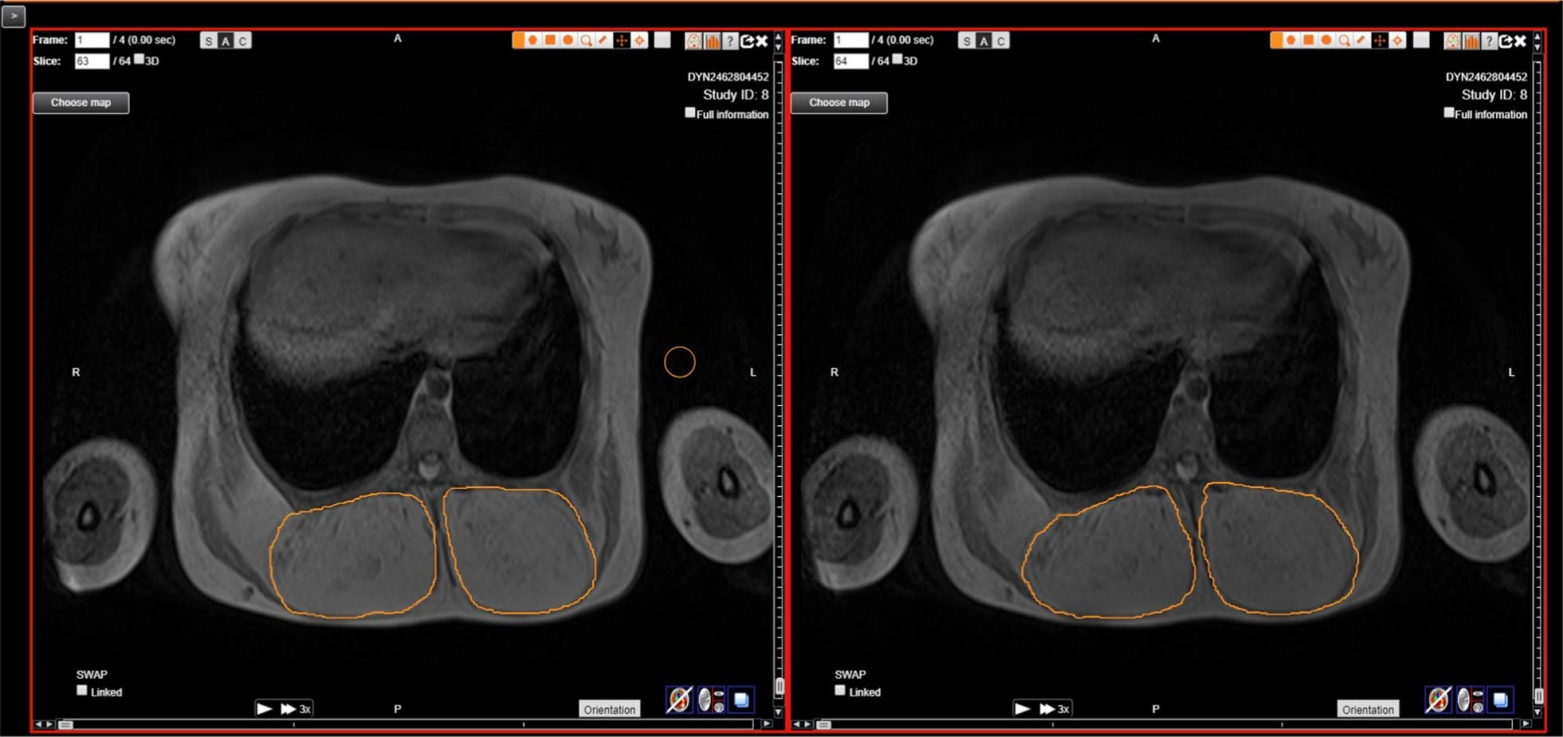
MRI of pelvis showing highlighted regions of interest (ROI) on adjacent slices, pre-treatment (left) and post 24 months treatment (right) (patient one)
